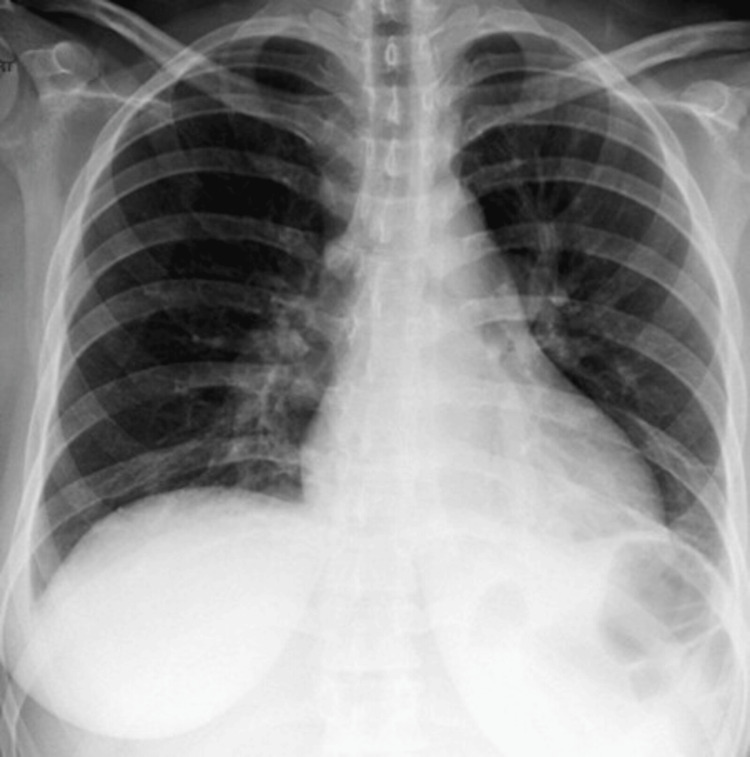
Portable chest radiograph demonstrating no acute cardiopulmonary abnormality, with no focal consolidation or pneumothorax.
